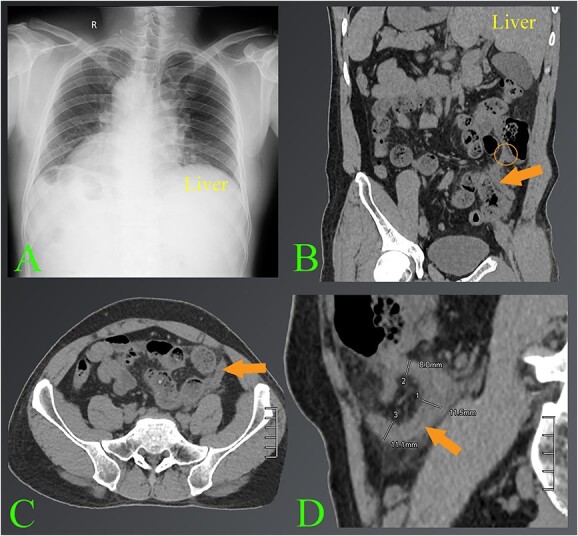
The images of appendicitis in a patient with situs inversus. (A) Chest X-ray of the chest shows that the heart is on the right side. (B–D) The CT images show the left-sided acute appendicitis with surrounding fatty infiltration (arrows). The root of the appendix located high in the left lumbar position is also seen (circle). Note where the liver is located on the left side.
